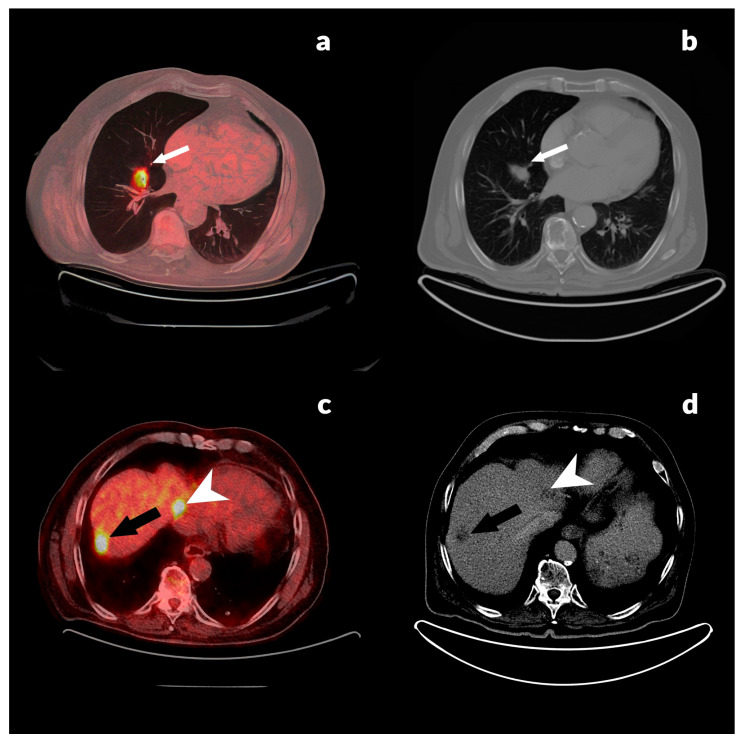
(a) PET/CT axial image detects a focal area of increased FDG uptake in the right pulmonary lobe, S4, SUVmax 7.50 (white arrow), corresponding to lung metastasis, (b) diagnostic CT scan in axial plane confirms a lesion in the right pulmonary lobe, S4 (white arrow), consistent with a metastatic lesion in the lung, (c) PET/CT axial image shows two hypermetabolic lesions in the liver in S8, SUVmax 8.9 (black arrow) and in S4a, SUVmax 8.0 (arrowhead), corresponding with liver metastases, and (d) diagnostic CT in axial plane shows two heterodense dominantly hypodense lesions in S8 (black arrow) and S4a (arrowhead), consistent with distant metastases in the liver.
